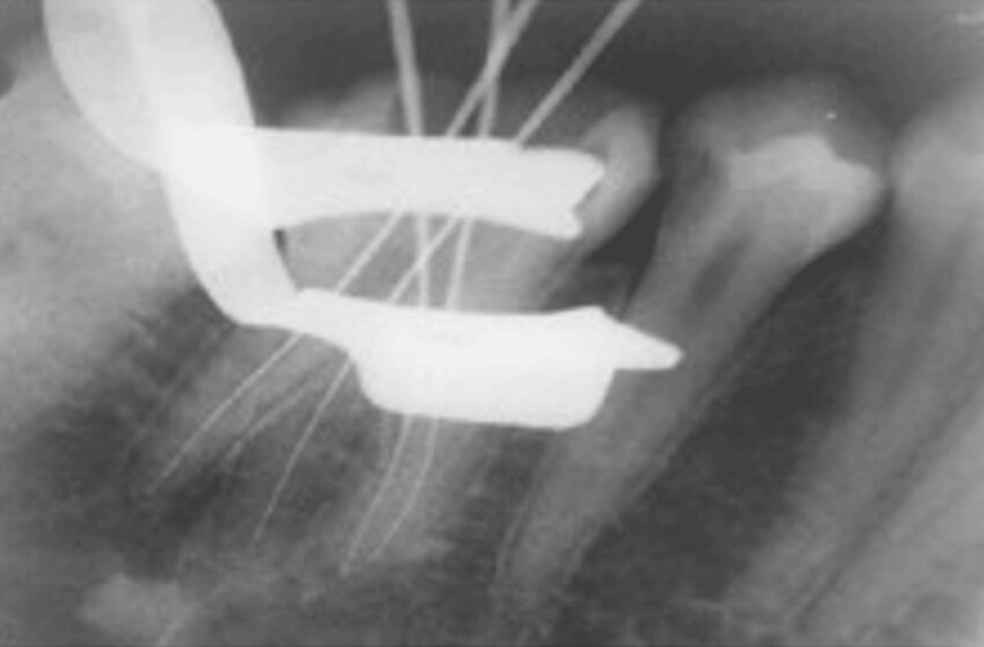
Working length radiograph
